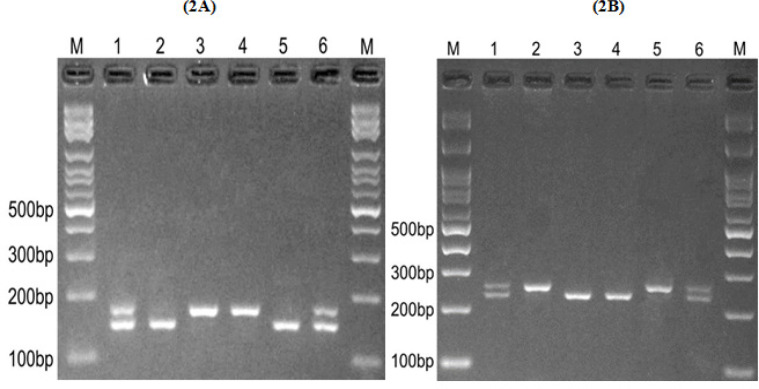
(2A). Electrophoresis pattern of the PCR-RFLP method for the detection of *PD-1*rs2227982(C/T) polymorphism. M: DNA marker; Lanes 1 and 6: CT; Lanes 2 and 5: CC; lanes 3 and 4: TT. (2B) electrophoresis pattern of the PCR-RFLP method for the detection of *PD-L1*rs2890658(C/A) polymorphism. M: DNA marker; Lanes 1 and 6: CA; Lanes 2 and 5: AA; lanes 3 and 4: CC
